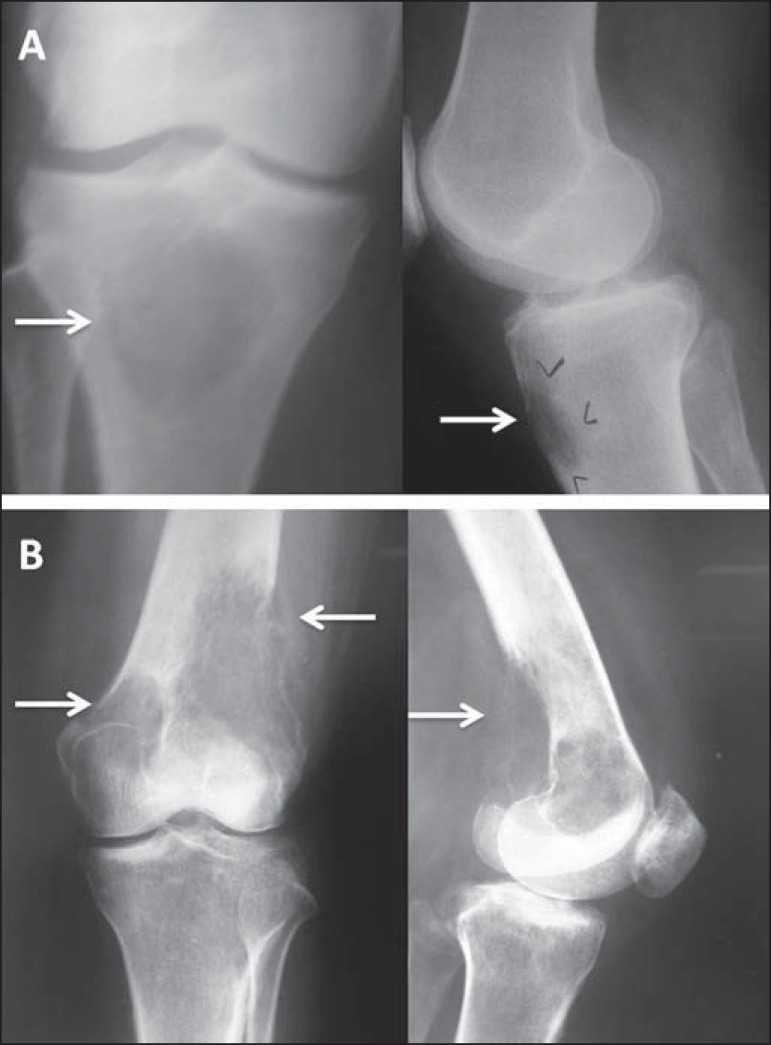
A: Anteroposterior and lateral radiography of the right knee
